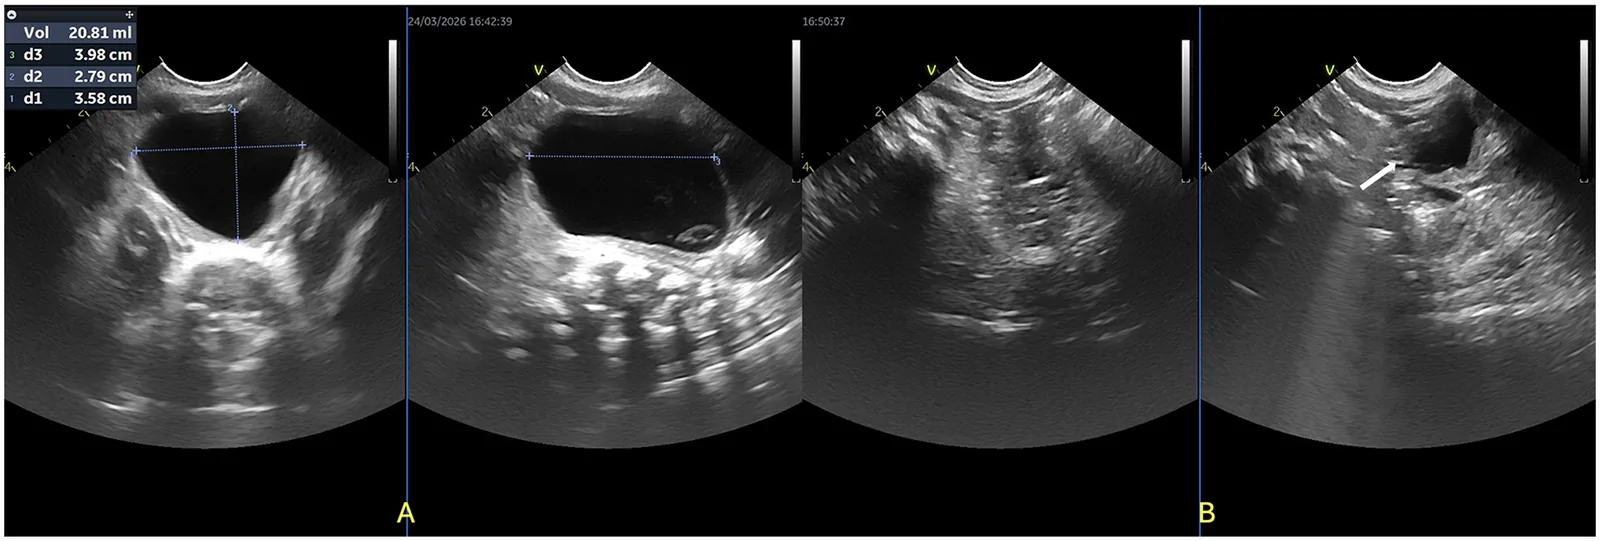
(A) Bladder POCUS (transverse and longitudinal views) demonstrating a well-filled urinary bladder, with an estimated volume of approximately 20 mL. (B) Bladder ultrasound demonstrating a collapsed urinary bladder following catheterization, with the urinary catheter *in situ* (arrow).
